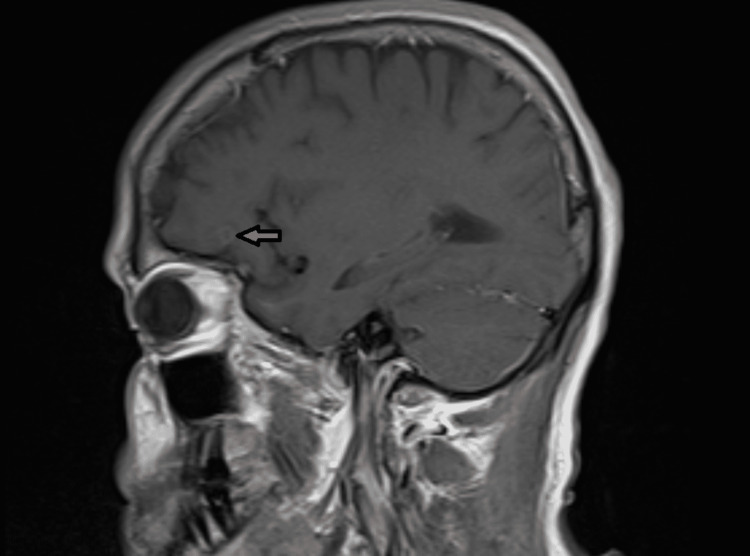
Sagittal T1-weighted magnetic resonance imaging demonstrating interval improvement in the size and intensity of the ring-enhancing lesion in the left inferior frontal cortex.
